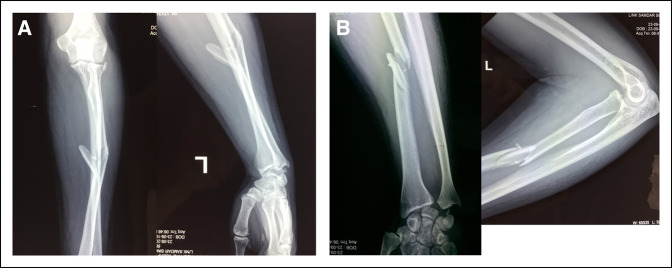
A, Preoperative radiographs showing the anteroposterior view. B, Preoperative radiographs showing the lateral view.
